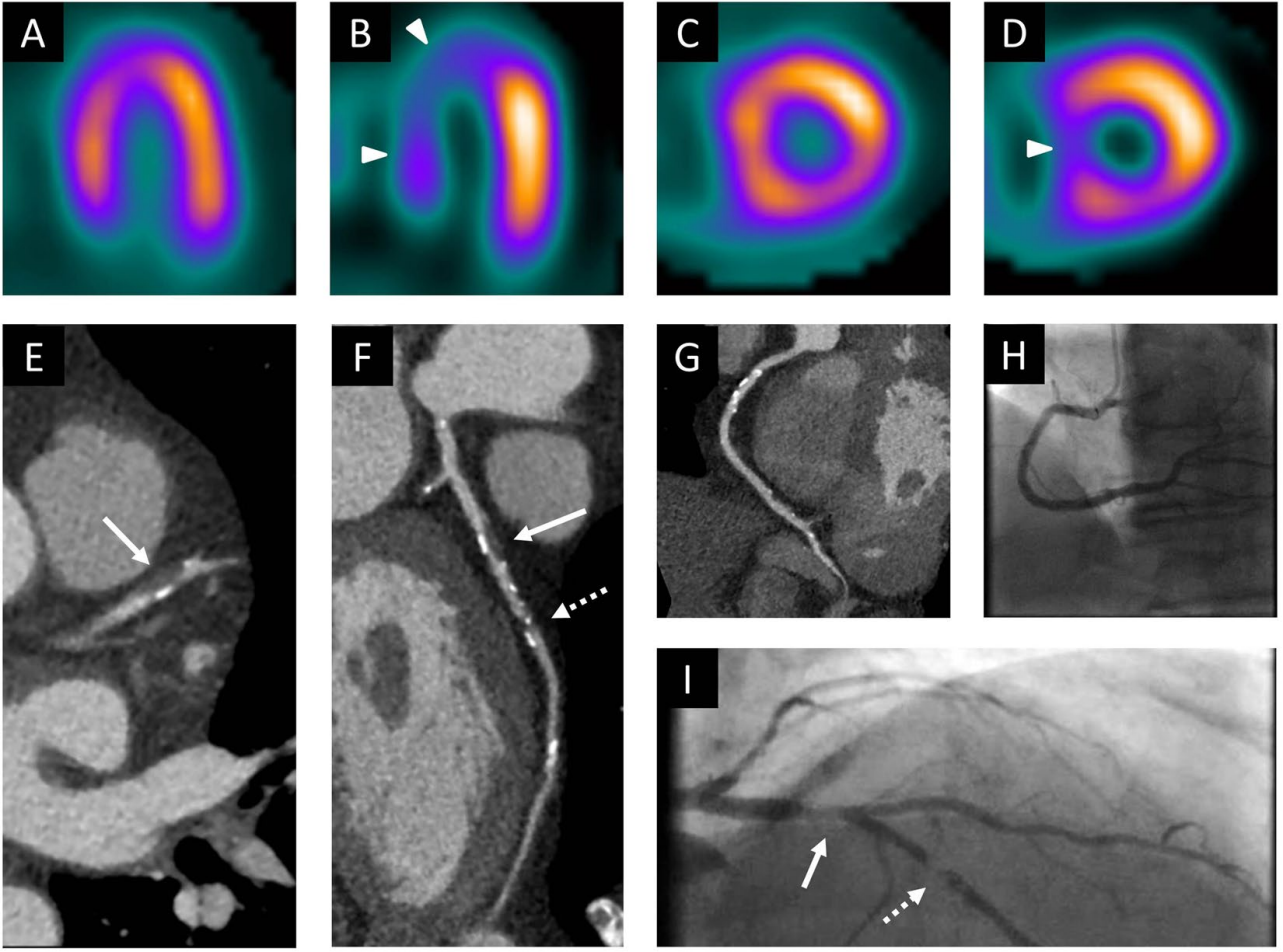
Example of a true positive CCTA examination. This 60 year old male patient underwent stress nuclear myocardial perfusion imaging because of recurrent chest pain. Stress images in the vertical short axis (B) and horizontal short axis views (D) are suggestive of decreased perfusion to the anterior, septal and apical portions of the left ventricular myocardium (arrowheads) and are largely reversible at rest (A,C). The examination was thus interpreted as concerning for ischemia involving the anteroseptal wall and the cardiac apex. At CCTA, transverse section (E) and curved multiplanar reformat (F) of the left anterior descending artery demonstrate a severe proximal stenosis caused by a predominantly non-calcified plaque (arrow). Another severe stenosis with mixed plaque is noted further distally (dotted arrow). The right coronary artery (G) and the circumflex artery show diffuse calcified and non-calcified plaque but no ≥50% stenosis. Absence of critical stenosis in the right coronary artery was confirmed on the catheter angiogram (H). The angiogram also confirmed the two critical stenoses in the left anterior descending artery (I). Both lesions were successfully treated with angioplasty and stent placement. CCTA = coronary computed tomography angiography.
